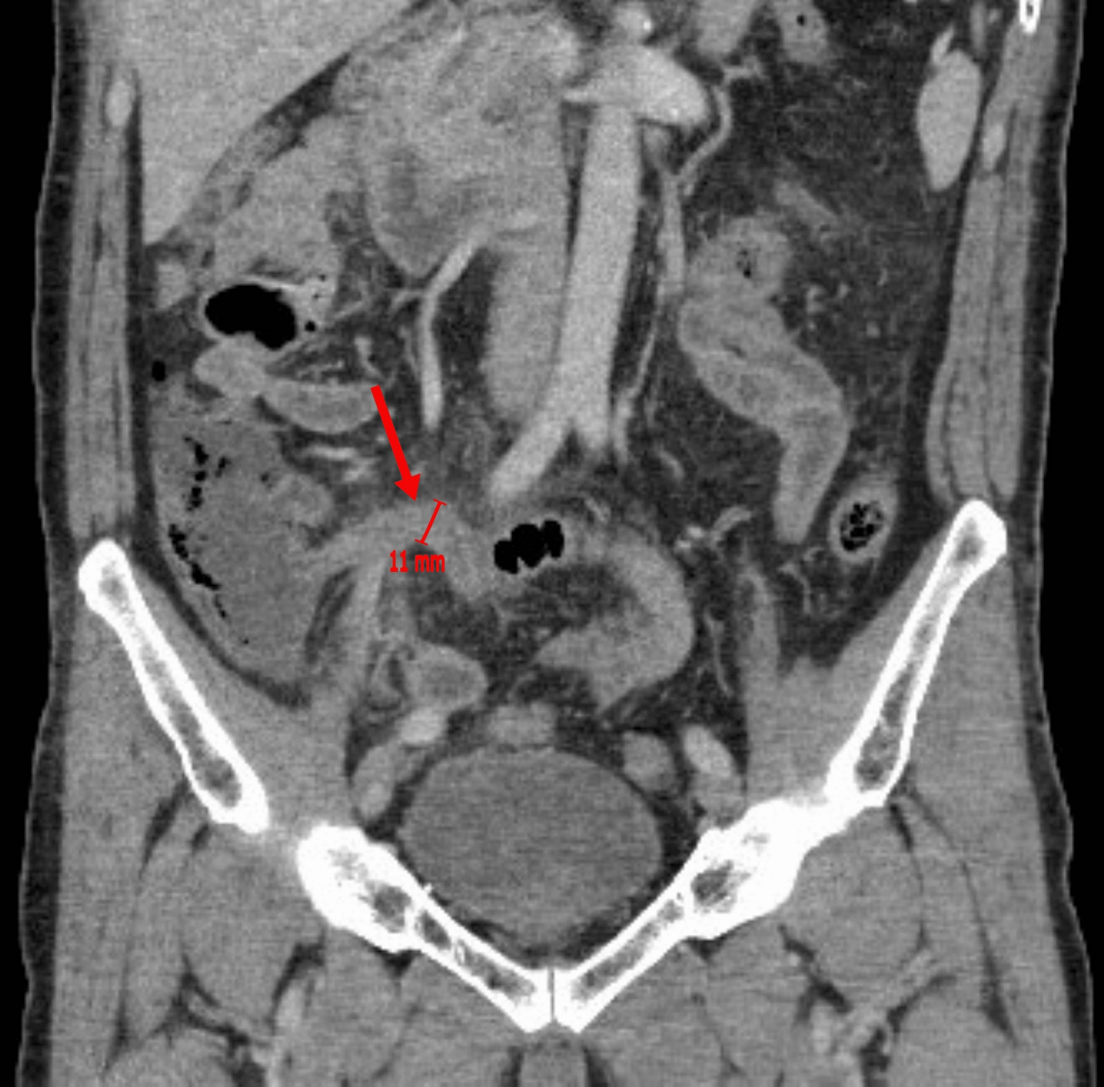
The preoperative CT abdomen/pelvis shows findings consistent with acute appendicitis without radiological evidence of perforation, revealing a dilated appendix (11 mm) labeled with an arrow.
